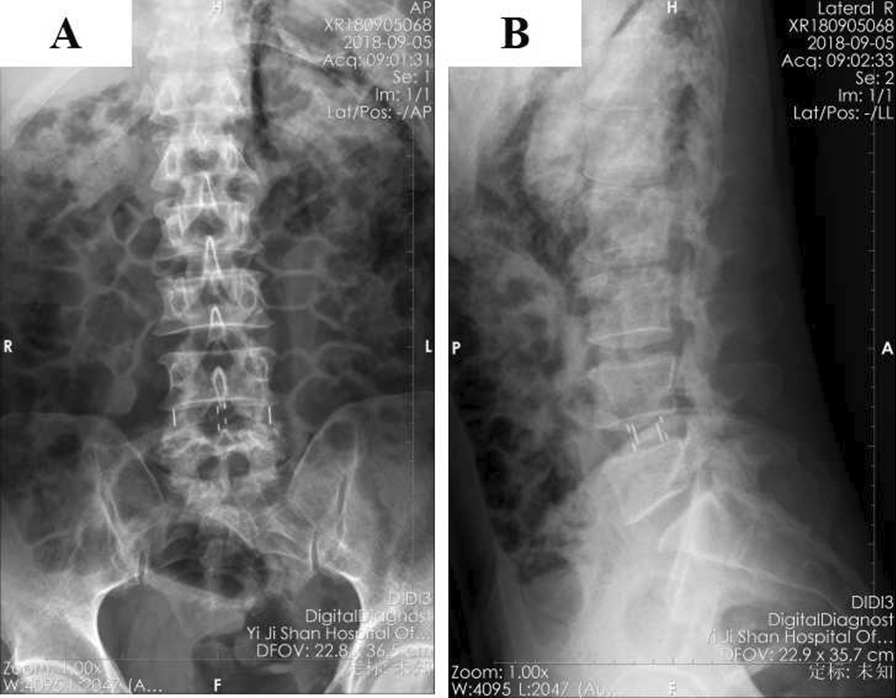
Positive (A) and lateral (B) X-rays of the lumbar spine after stand-alone OLIF surgery
